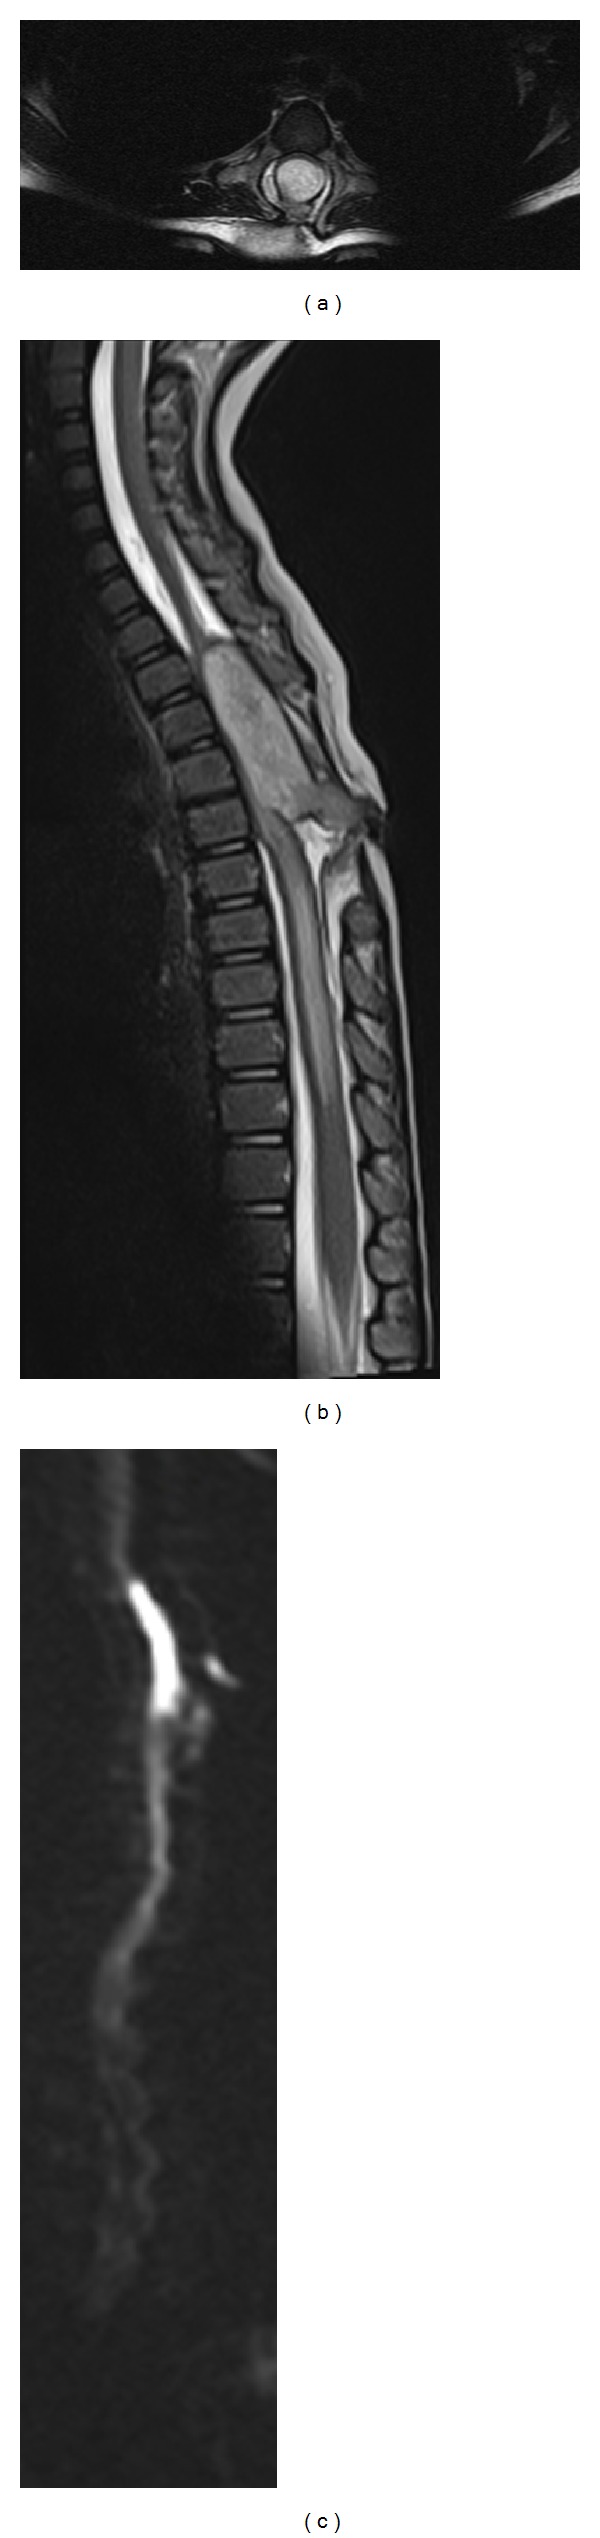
Axial T2-W (a), sagittal T2-W (b), and DW (c) MR images. (a) A hyperintense well-defined, round, intramedullary lesion is seen spanning T3–T6 vertebral levels. (b) The hyperintense lesion demonstrates continuity with the skin surface consistent with a dorsal sinus tract. (c) There is hyperintensity of the spinal lesion on the sagittal DW image consistent with restricted water diffusion.
